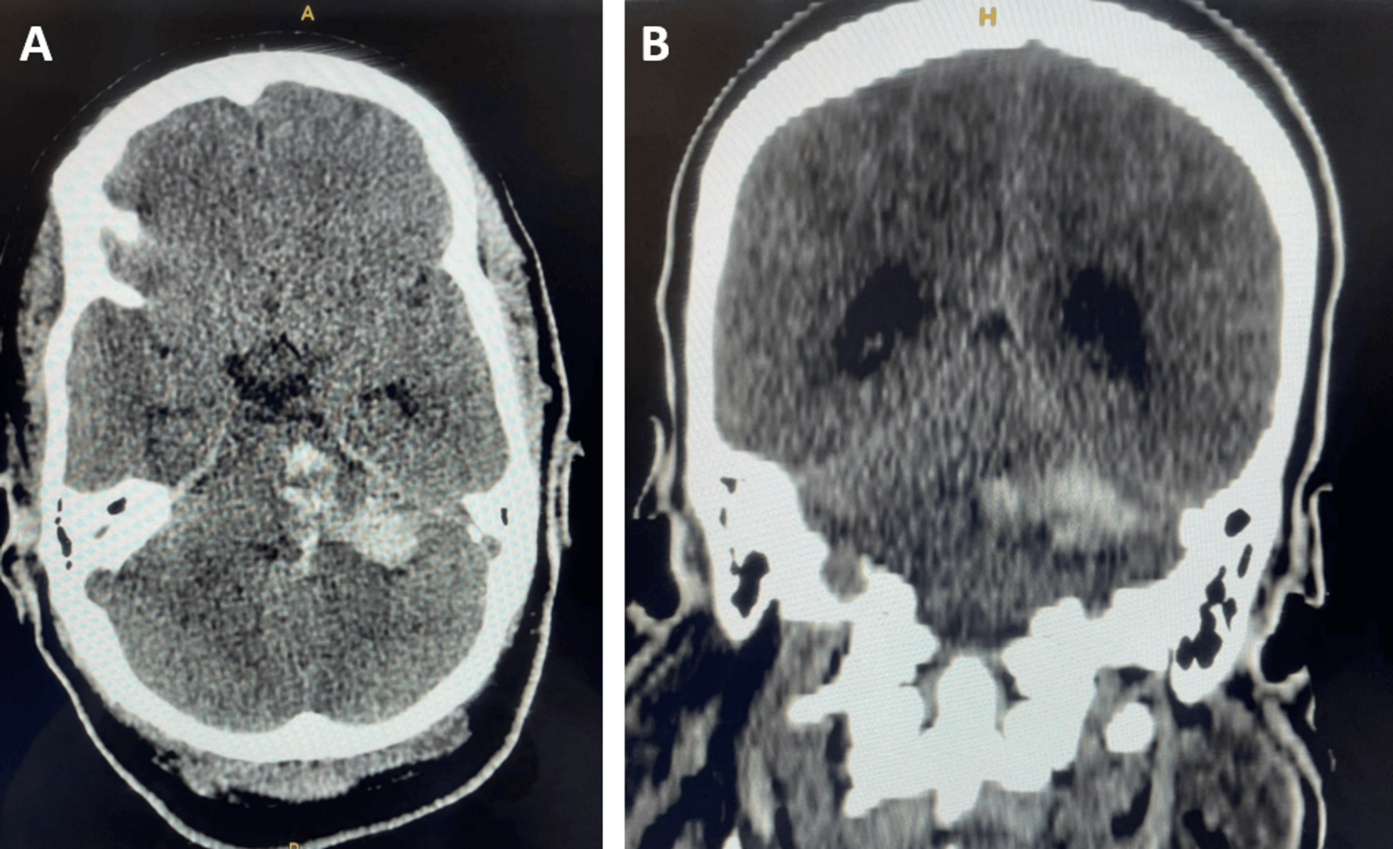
Non-contrast brain CT. Images are demonstrating an intraparenchymal brainstem hematoma extending to the left cerebellar hemisphere and left thalamus, with mass effect on the fourth ventricle, shown on (A) axial images (B) and coronal reconstruction.
